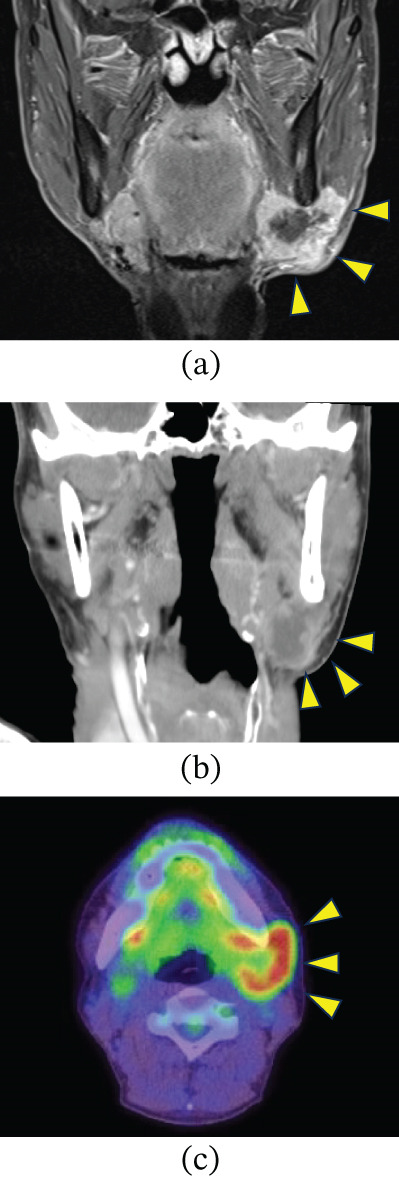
Radiological findings at the initial presentation. (a) Contrast‐enhanced CT showing a mass lesion with internal low‐attenuation areas in the left submandibular region, without mandibular destruction or resorption. (b) Contrast‐enhanced MRI showing an irregularly marginated mass replacing the left submandibular gland, with heterogeneous internal signal intensity and predominantly peripheral heterogeneous enhancement. (c) FDG‐PET/CT demonstrating increased uptake in the left submandibular mass (maximum standardized uptake value, 9.2), without evidence of another primary malignancy or distant metastasis.
